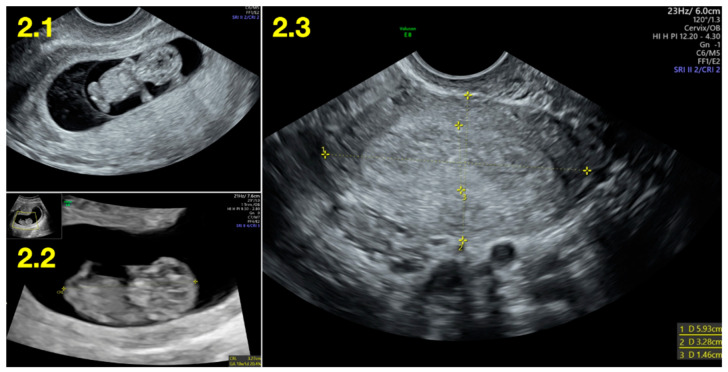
Transvaginal ultrasonography images at 10 weeks and 1 day of pregnancy. (2.1,2.2). Left uterus with a gestational sack and fetus at 10 weeks and 1 day of intrauterine development, Crown-Rump Length (CRL) was 3.27 cm. (2.3) Right uterus: distance 1 (1D on image)—uterus length 5.93 cm; distance 2 (2D on image)—uterus height 3.28 cm; distance 3 (3D on image)—endometrial thickness 1.46 cm.
